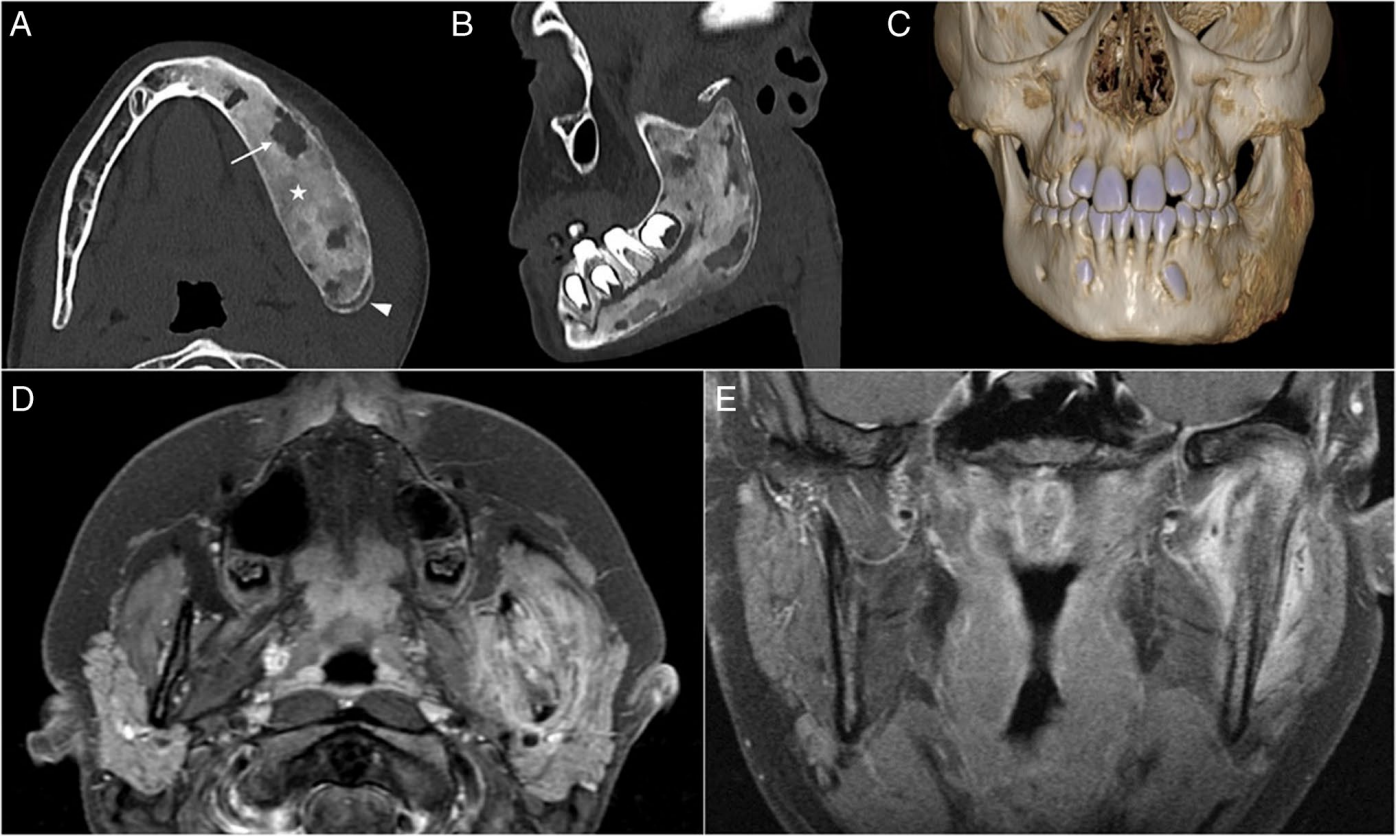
Imaging characteristics of mandibular lesions on computed tomography (CT) and magnetic resonance (MR): images obtained from an 8-year-old girl (a–c) and from a 14-year-old girl (d, e). a–c Axial (a), coronal (b), and three-dimensional reconstructed (c) CT images of the mandible reveal an extensive lesion of the left mandibular body and ramus, with bone expansion, heterogeneous ground-glass density (*asterisk*), lytic areas (*arrow*), cortical irregularity, and periosteal reaction (*arrowhead*). d, e Axial (d) and coronal (e) T1-weighted contrast-enhanced fat-saturated MR images of another patient show severe inflammatory changes of the soft tissues surrounding the left mandibular ramus
